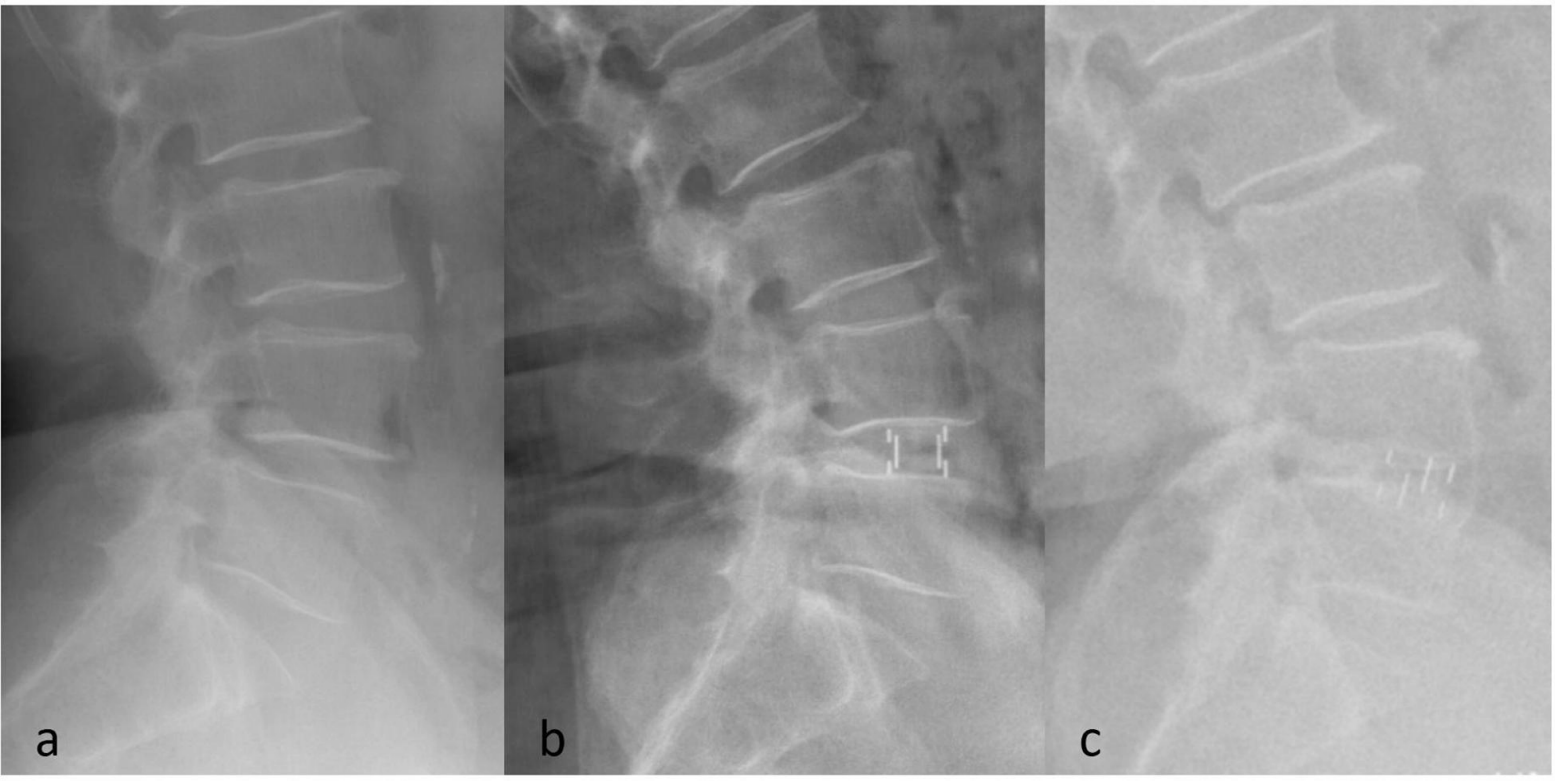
Preoperative (a), postoperative (b), and last follow-up (c) radiographs of SA-OLIF.
